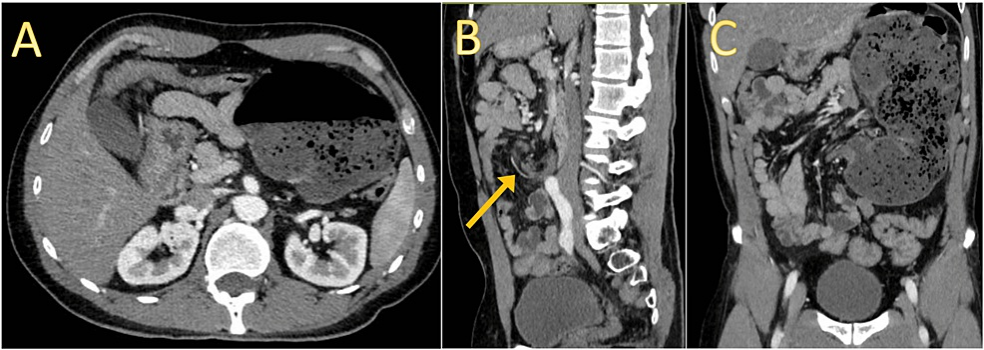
Abdominal contrast-enhanced CT. (A) Axial view in the arterial phase, the cecum is dilated (measured at 10.6 cm) in the left hypochondrium. (B) Sagittal view where the twisting of the mesentery is visible (yellow arrow). (C) Coronal view with congestion of the mesentery and fat stranding.
